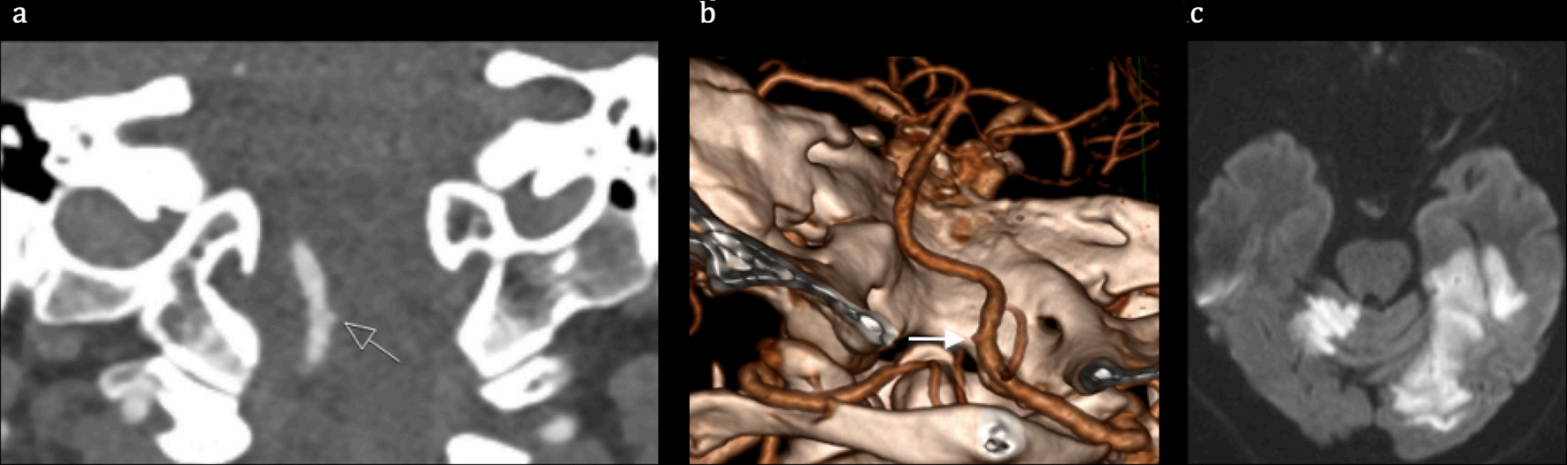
Neuroimaging (CTA, Diffusion Weighted MRI) following acute neurological symptoms. Coronal (a) and 3D posteroanterior CTA (b) demonstrating subtle irregularity of the right V4 VA. DWI demonstrating acute ischaemic lesions in the right cerebellum, left parahippocampal and fusiform gyri and left occipital lobe (c).
